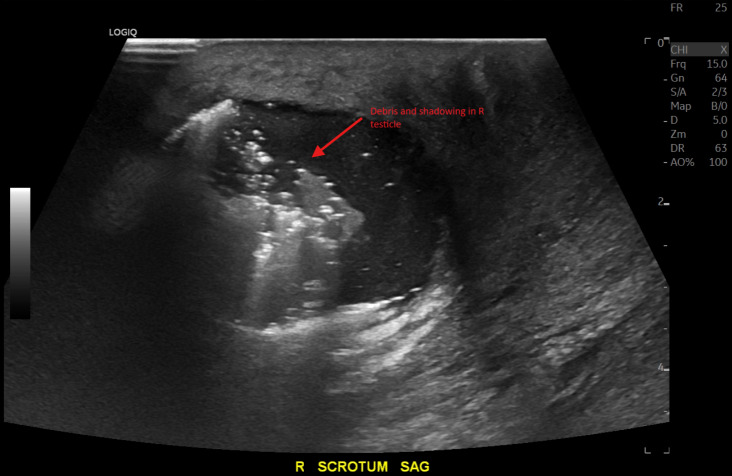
Point of care ultrasound (POCUS) (sagittal view) of the right hemiscrotum demonstrates shadowing and debris. R, right; SAG, sagittal.
